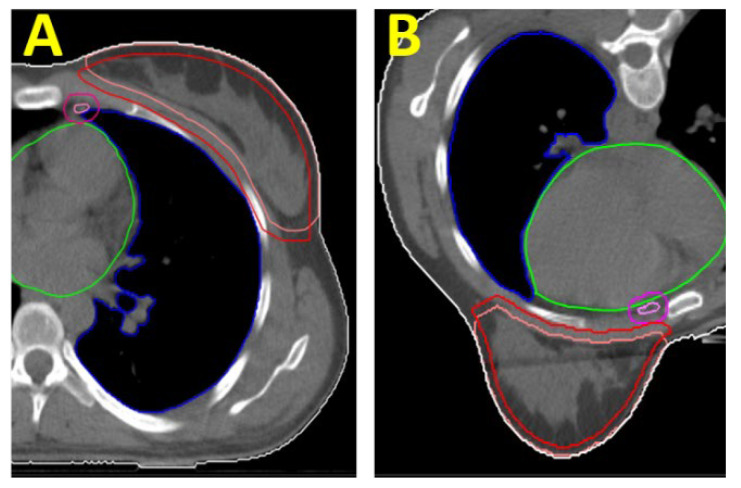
CT sections of a patient with left-sided breast cancer: (A) in the supine and (B) in the prone positions. Contours marked are the CTV “B” (light red), the PTV “B” (red), the CTV “PSR” (pink), and the PTV “PSR” (purple). In addition, the OAR “heart” (green) and OAR “left lung” (blue) are depicted.
